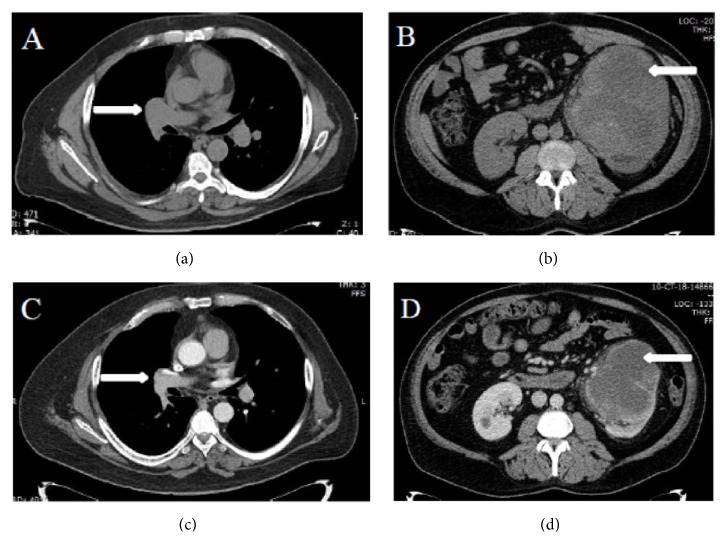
CT CAP*. (a) and (b) Axial images of CT CAP showed right sided hilar lymphadenopathy and a large left kidney mass. (c) and (d) Axial images of CT CAP showed improvement in right sided hilar lymphadenopathy and reduction in size of left kidney mass, after 8 weeks of therapy with cabozantinib.
